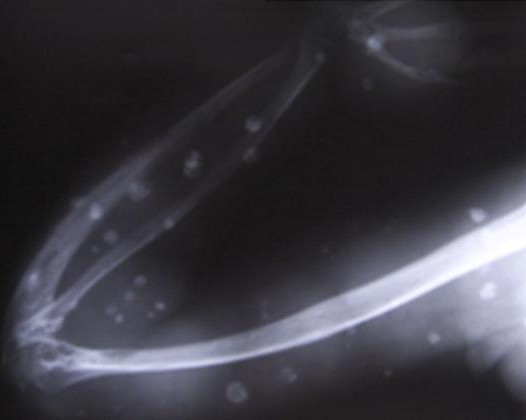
X-ray showing multiple soft-tissue swellings, hypotrophy of the bone, and multiple well-defined, radio-opaque lesions consistent with phleboliths.
